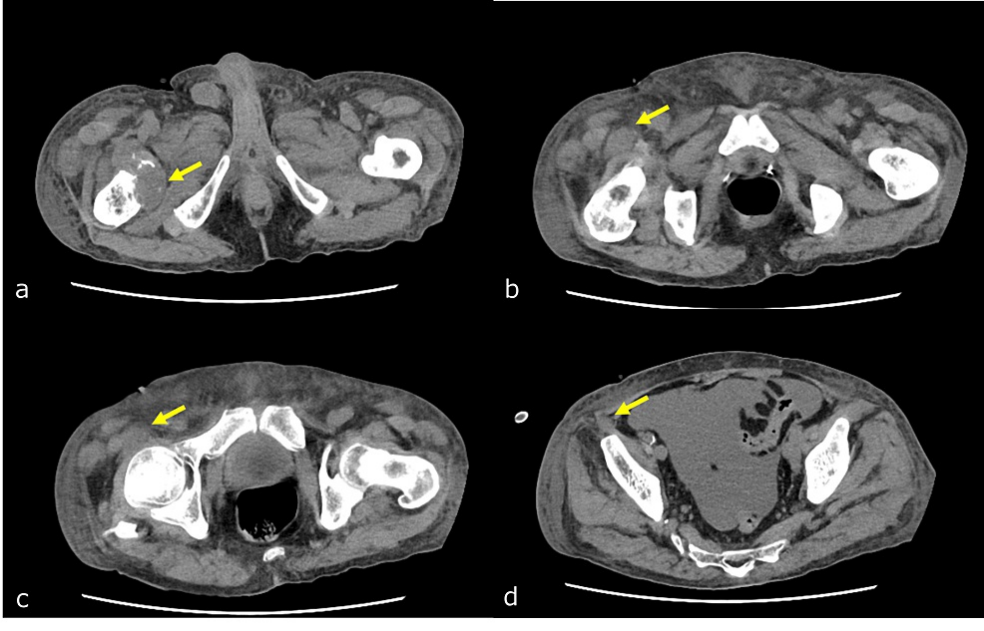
CT scan of the pelvic region one month after radiation therapy The size of the bone metastasis in the right femoral lesser trochanter remained similar before radiation therapy (a, indicated by the yellow arrow). However, there was an improvement in the infiltration and muscle thickening near the right iliopsoas muscle (b-d, indicated by the yellow arrow).
